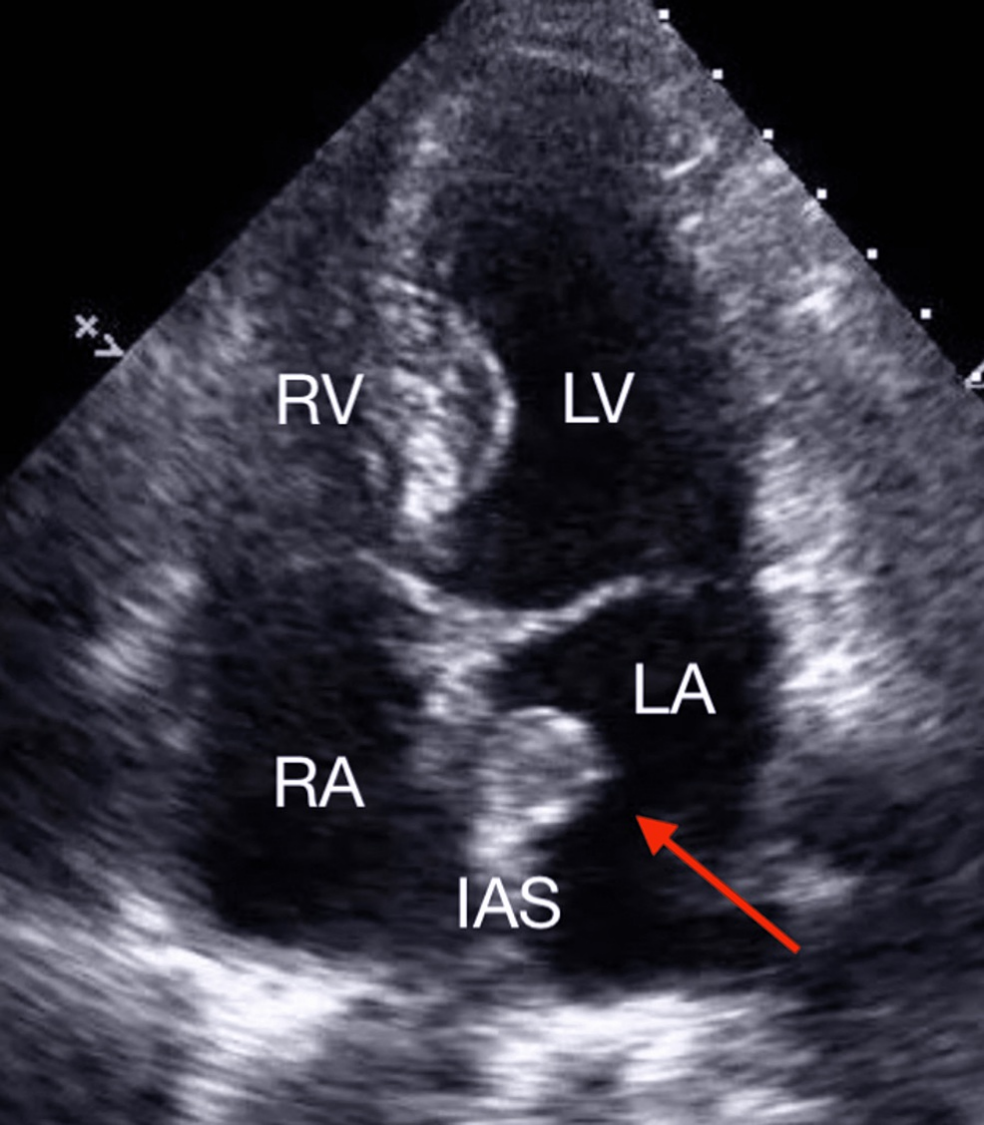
Apical Four-Chamber View of Atrial Myxoma Transthoracic echocardiogram showing a 2x2.3 cm heterogenous mass (arrow) protruding from a thickened interatrial septum into the left atrium RA = right atrium; LA = left atrium; RV = right ventricle; LV = left ventricle; IAS = interatrial septum
